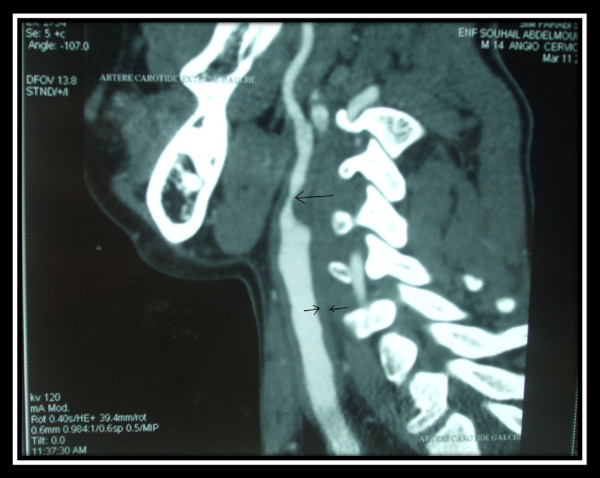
Cervical CT angiography shows irregular thickening of the left common carotid artery wall.
